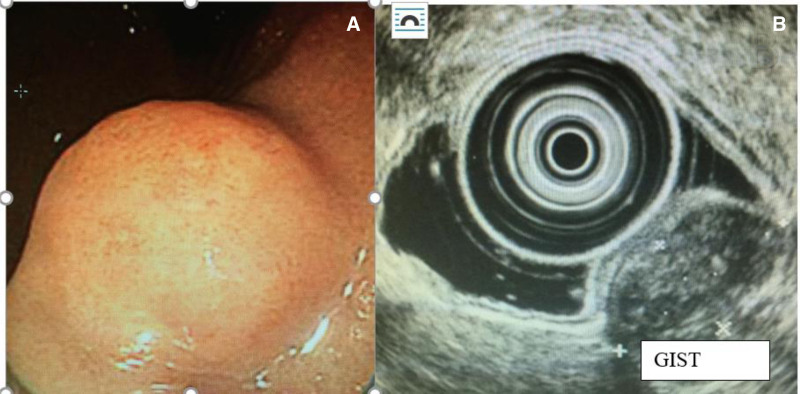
Sixteen-year-old with chronic abdominal pain. (A) Gastric submucosal lesion seen in the stomach on EGD. (B) EUS with GIST. EGD = esophagogastroduodenoscopy; EUS = endoscopic ultrasound; GIST = gastrointestinal stomal tumor.
